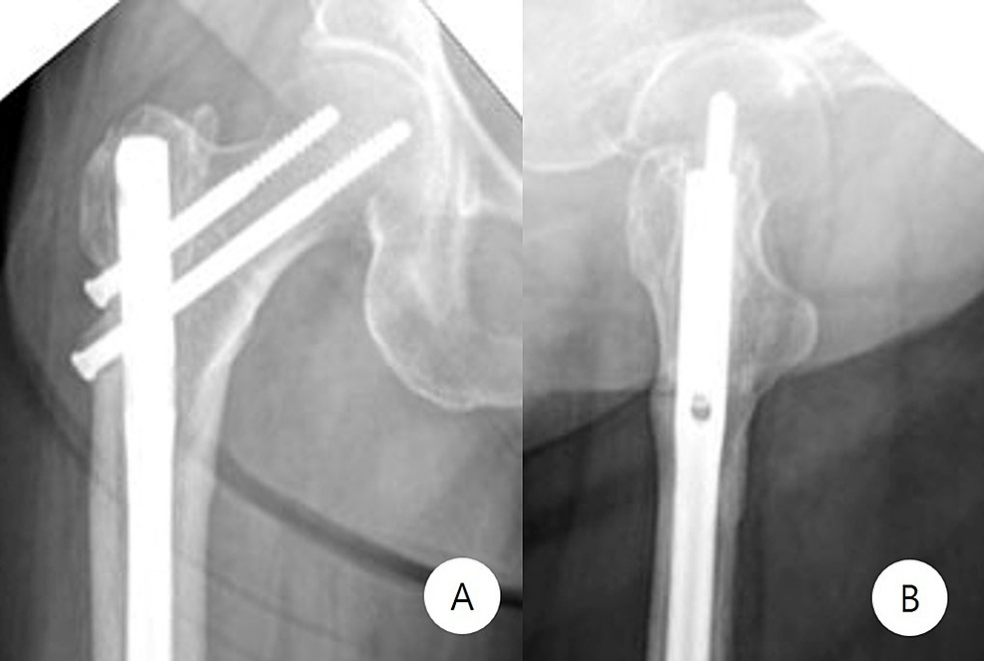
Follow-up imaging - case 2 Six-month follow-up anteroposterior (A) and lateral (B) radiographs show complete healing of the subtrochanteric fracture
